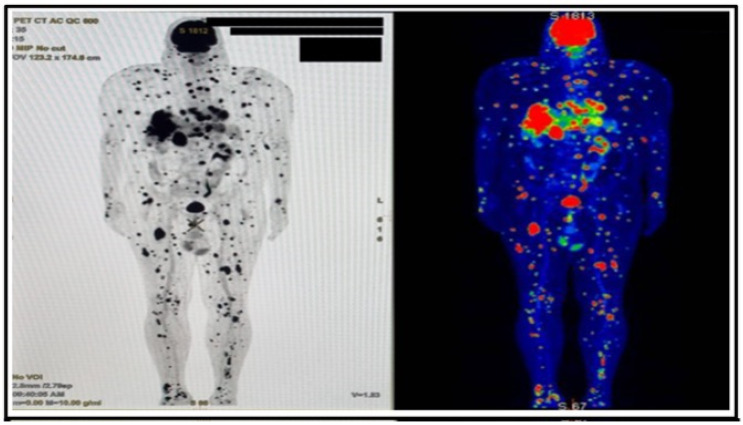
PET/CT demonstrates multiple hypermetabolic lesions, primarily localized in the liver, pancreas, and lungs, with additional findings suggestive of cardiac hypermetabolic involvement.
